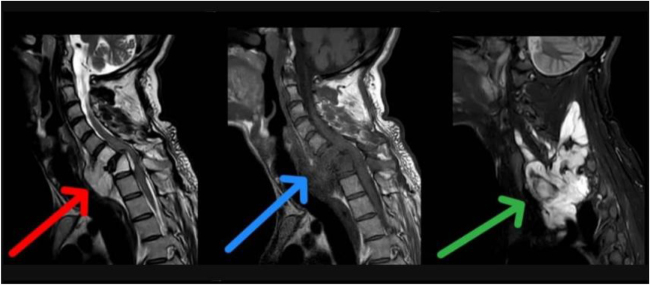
Sagittal MRI scan shows the lesion. T2 (red) T1 (blue) STIR (green).
